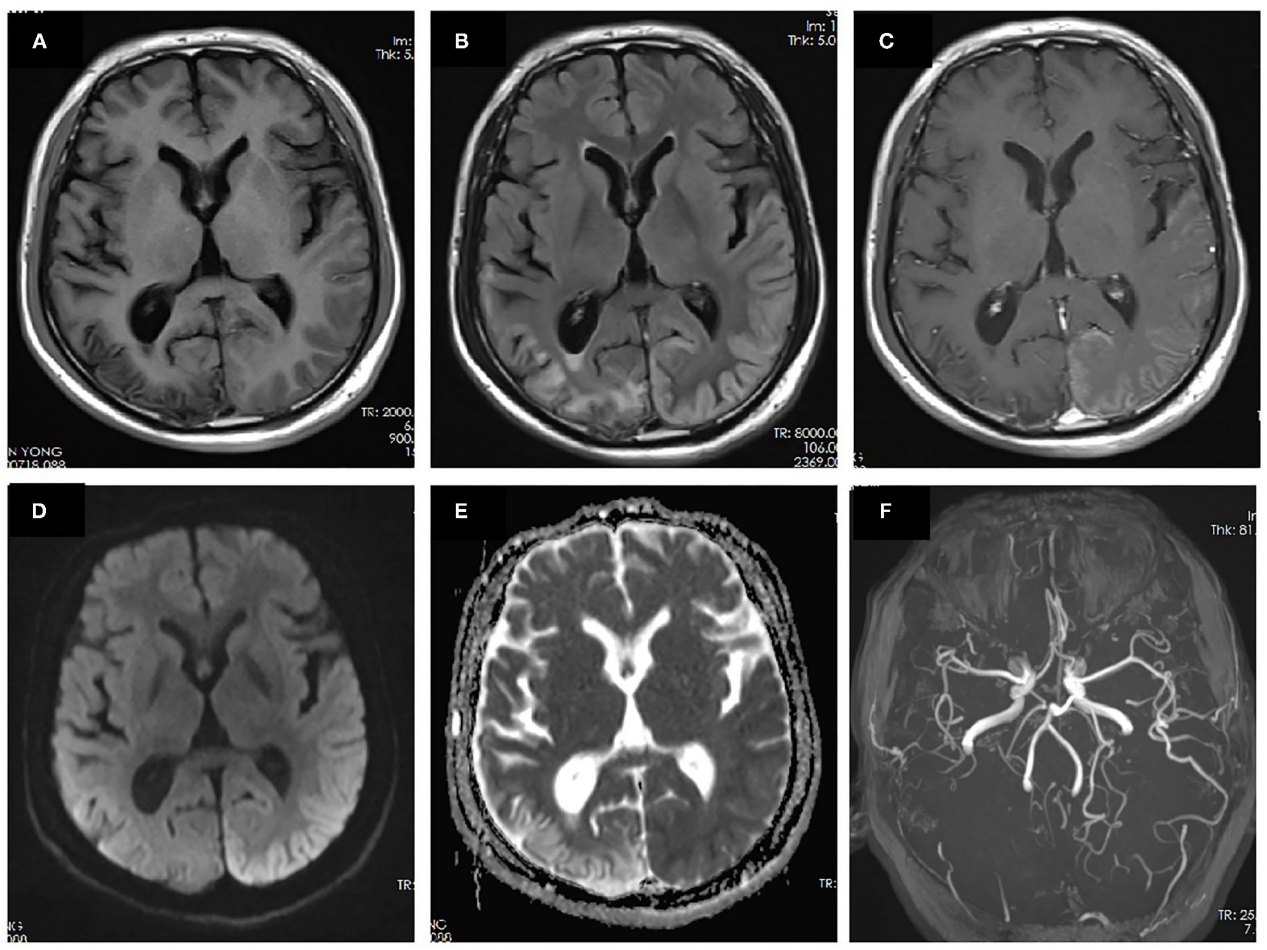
MRI performed 10 days after onset of the second prolonged attack. Right temporal-occipital hyperintensity on T1-weighted images (A) with gliosis on T2-FLAIR images (B) suggests the chronic stage of cortical necrosis caused by first prolonged attack. Left temporal–occipital cortex hyperintensity on T2-FLAIR images (B) with gyriform enhancement (C), restricted diffusion (D), and normal apparent diffusion coefficient (ADC) (E) suggests the subacute stage of cortical necrosis caused by second prolonged attack. MRA demonstrated vasodilation of the branches of the left middle cerebral artery (MCA) and posterior cerebral artery (PCA) (F).
